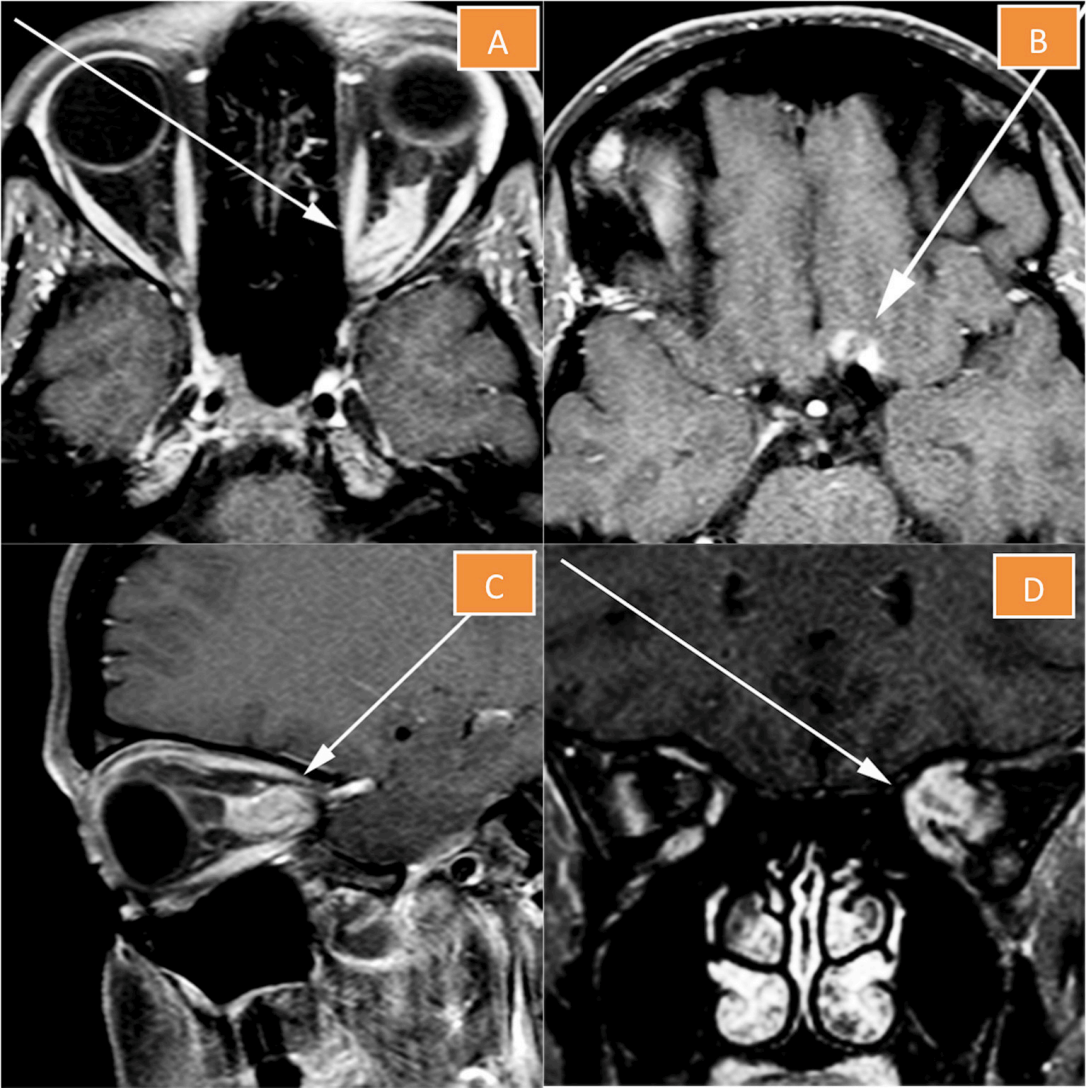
Magnetic resonance imaging of the left orbit. The lesion essentially replaces the optic nerve (A,C,D), and has a component extending superiorly onto the left side of the planum sphenoidale (B).
